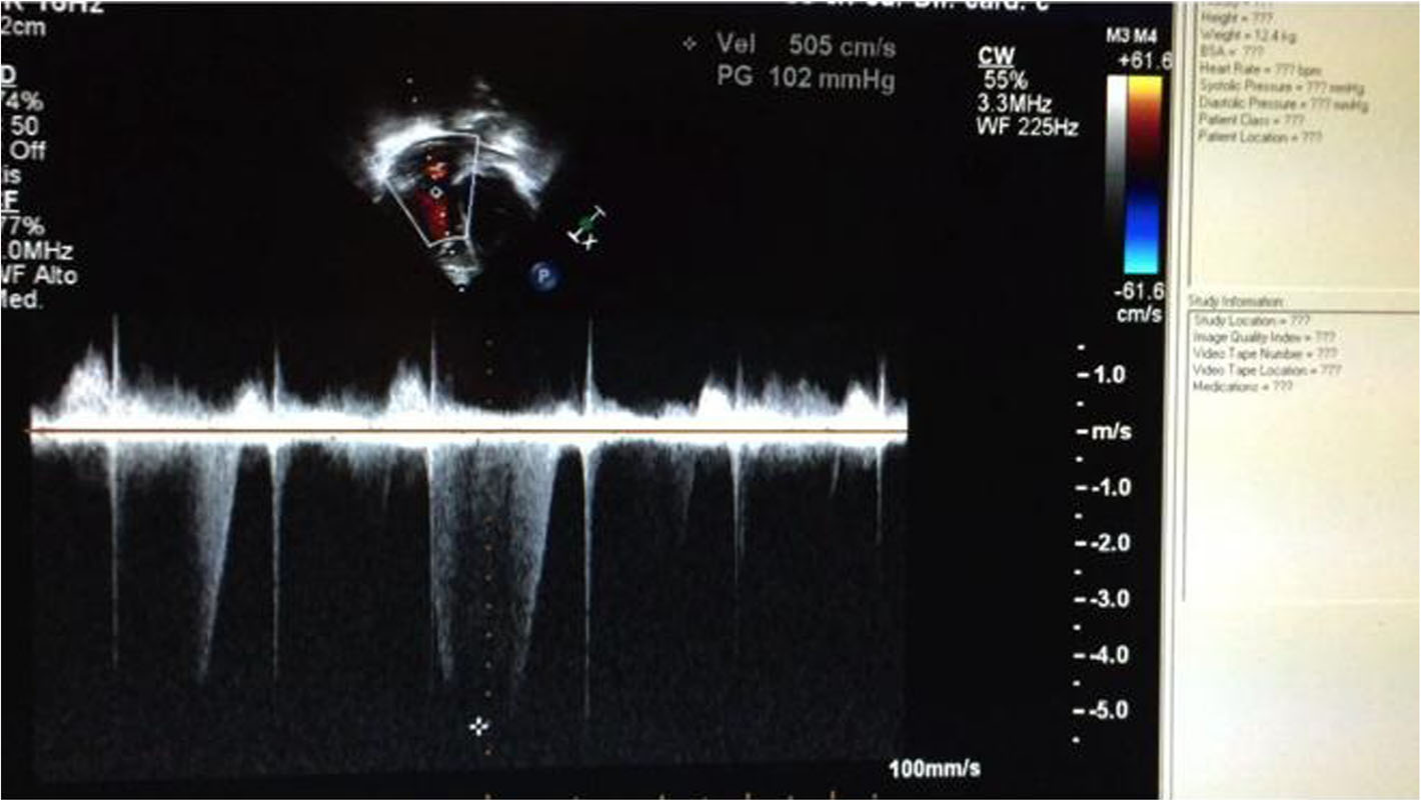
Four-chamber view. CW Doppler evidences high-velocity tricuspid insufficiency: 5.05 m/sec corresponding to pulmonary pressure of 107 mmHg
